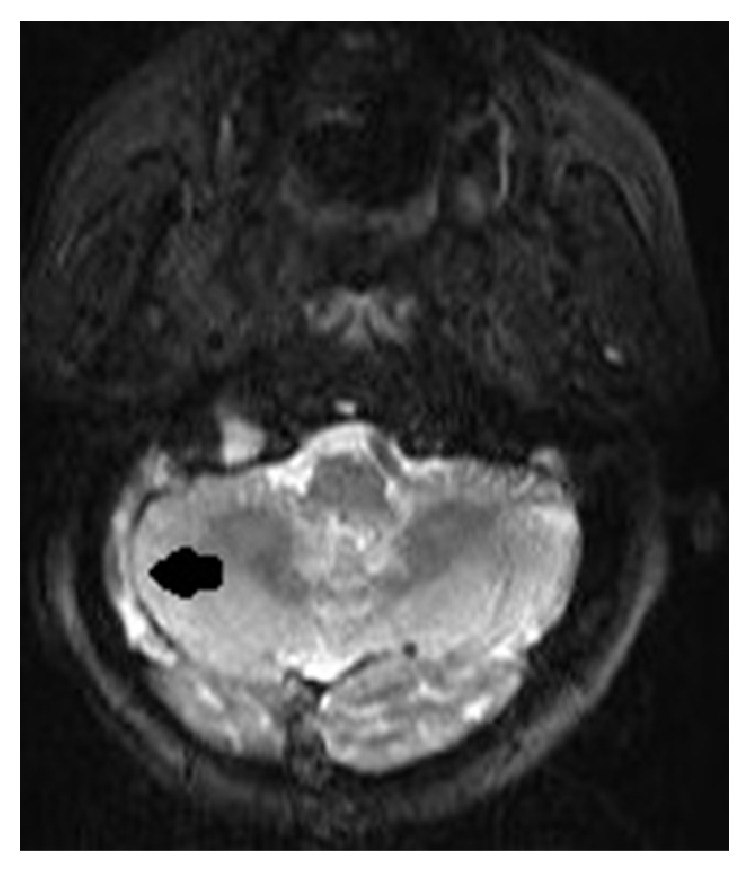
Axial T2-weighted MRI revealed signal void loss on the right sigmoid and transverse sinuses.
